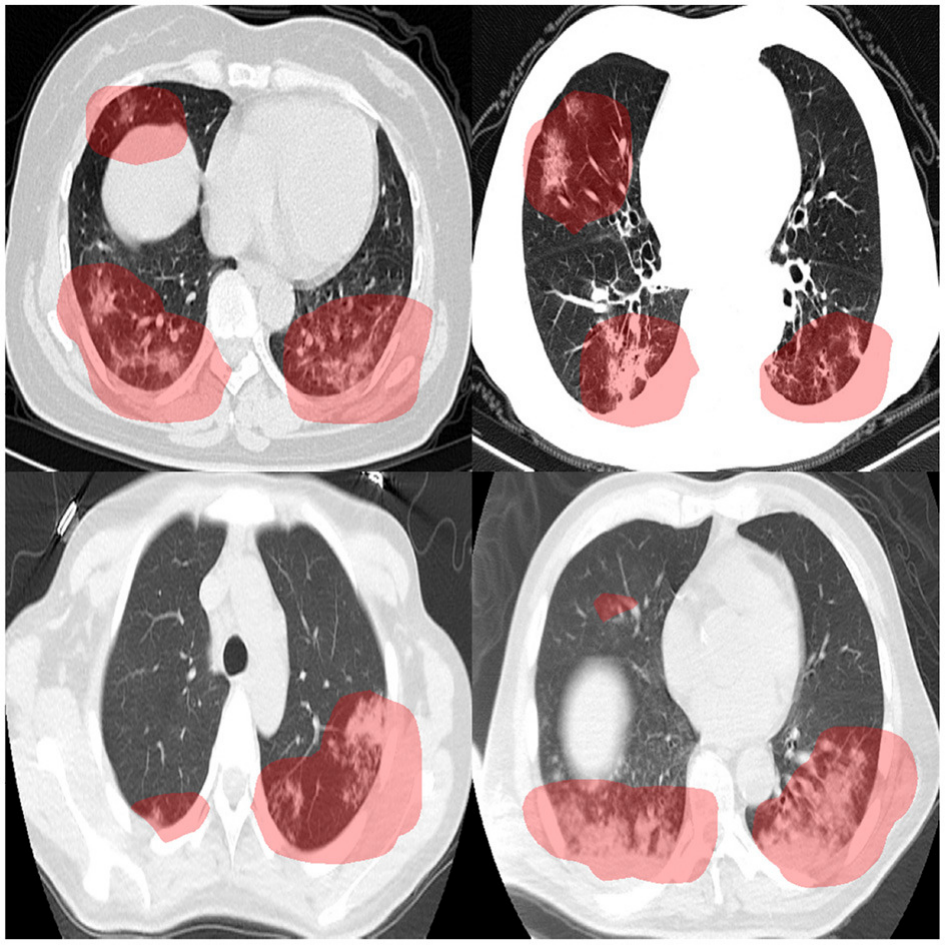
Example chest CT images from four COVID-19 cases reviewed and reported on by two board-certified radiologists, and the associated critical factors (highlighted in red) as identified by GSInquire (33) for COVID-Net CT-2 L. Based on the observations made by two expert radiologists, it was found that the critical factors leveraged by COVID-Net CT-2 L are consistent with radiologist interpretation.
